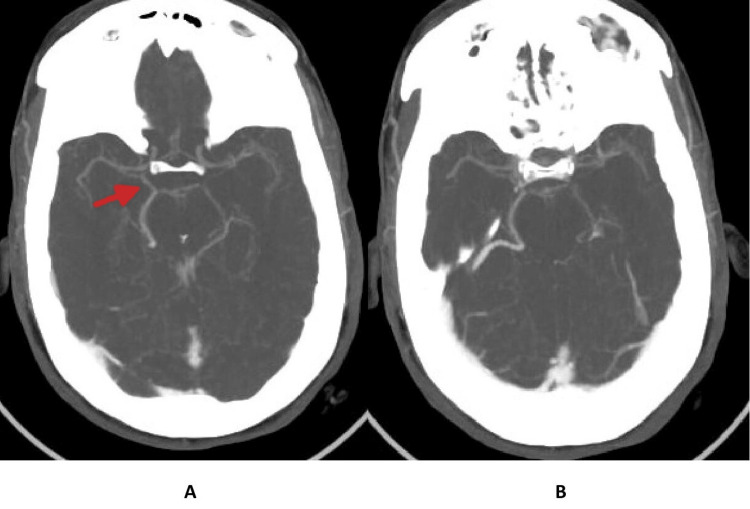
CT angiography showing acute sub-arachnoid hemorrhage with no evidence of aneurysm or vascular malformation. The circle of Willis and major intracranial arteries have normal contrast distribution without filling defects or aneurysmal dilation. There is minimal prepontine space pooling with slight residual right cavernous extension, consistent with a perimesencephalic pattern of subarachnoid hemorrhage (red arrow).
